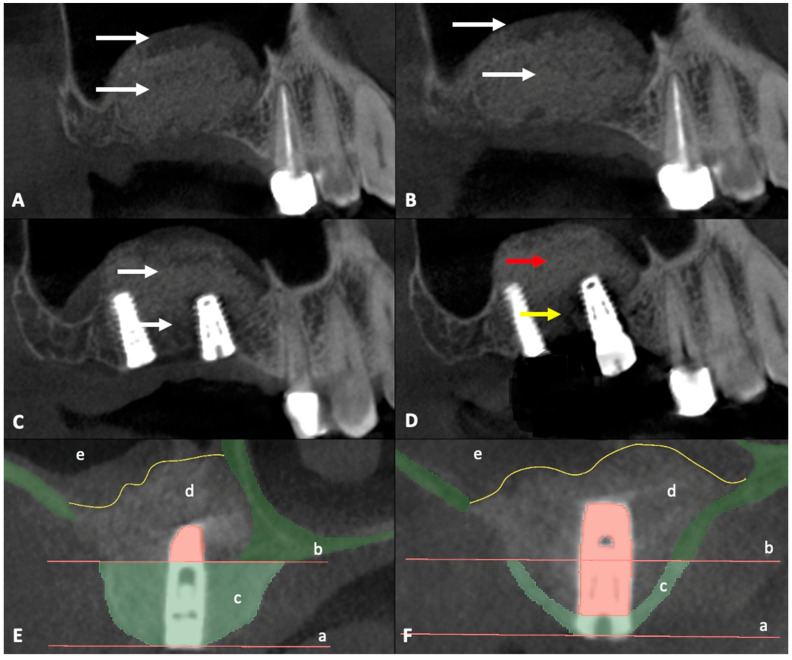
CBCT data obtained before and after surgery and ROI measurements with 3D Slicer image computing platform. (A) Postoperative CBCT image obtained after MSFA. No abnormalities were observed during the healing process. White arrow: Region of integrated graft material and an inflammation-free maxillary sinus. (B) Preoperative CBCT image obtained before the insertion of dental implants. No signs of pathology were present. (C) Postoperative CBCT image obtained after the insertion of dental implants. (D) Postoperative CBCT image obtained 8 months before implant placement. Red arrow: Homogeneous graft material, without evidence of infection or fistula formation. Yellow arrow: Region of non-integrated graft material lacking osseointegration. (E) ROI with sufficient bone availability. a: Alveolar crest; b: Inferior border of the lateral window; c: ROI; d: Xenohybrid bone graft; e: Sinus membrane; yellow line: Tachosil^®^ membrane; green part: autogenous bone. (F) ROI with limited bone availability. Labeling as in (E).
